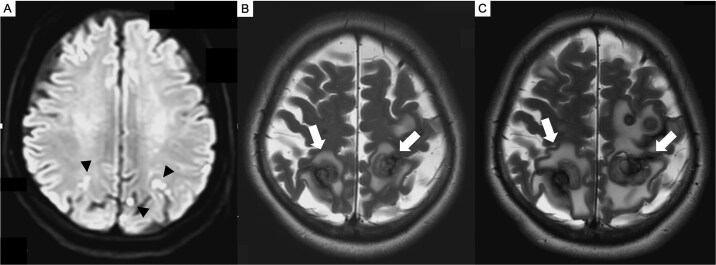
Brain MRI. (A) Preoperative diffusion-weighted imaging (DWI) showing multiple acute cerebral infarcts (arrowed) in the parietal lobe. (B) MRI (T2-weighted imaging) at 7 months postoperatively showing multiple new enhancing intracranial lesions (arrowed) corresponding to previous infarcts sites in the parietal lobe. (C) MRI (T2-weighted imaging) at 8 months postoperatively showing progressive enlargement and edema (arrowed).
